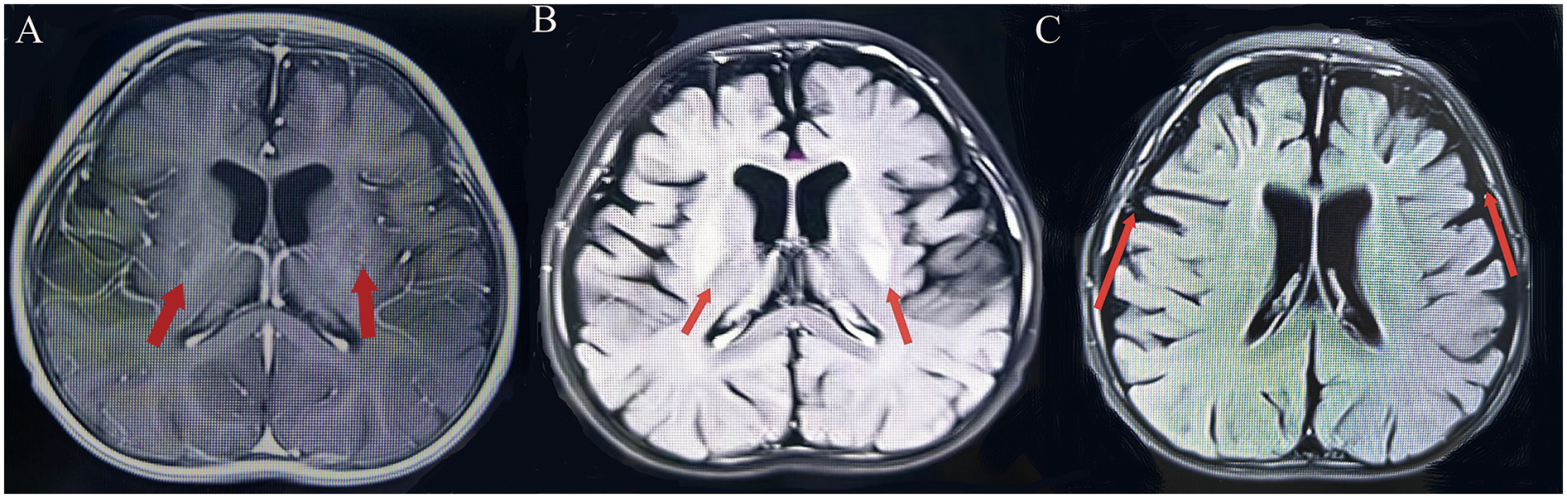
(Patient 1) Brain MRI. (A) T1-weighted image: symmetrical lenticular nucleus hyperintensity. (B) T2-weighted image: symmetrical lenticular nucleus hyperintensity. (C) Diffuse cerebral atrophy.
